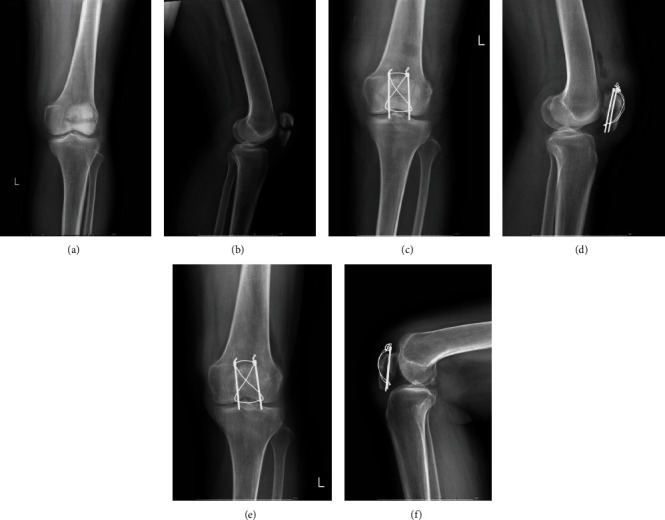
(a–f) A 54-year-old woman with a transverse patellar fracture had ring pin tension band fixation. (a) An anteroposterior X-ray shows the transverse patellar fracture after injury. (b) A lateral X-ray after injury. (c) A postoperative anteroposterior X-ray of 1 month. (d) A postoperative lateral X-ray of 1 month. (e) A postoperative anteroposterior X-ray of 6 months. (f) A postoperative lateral X-ray.
